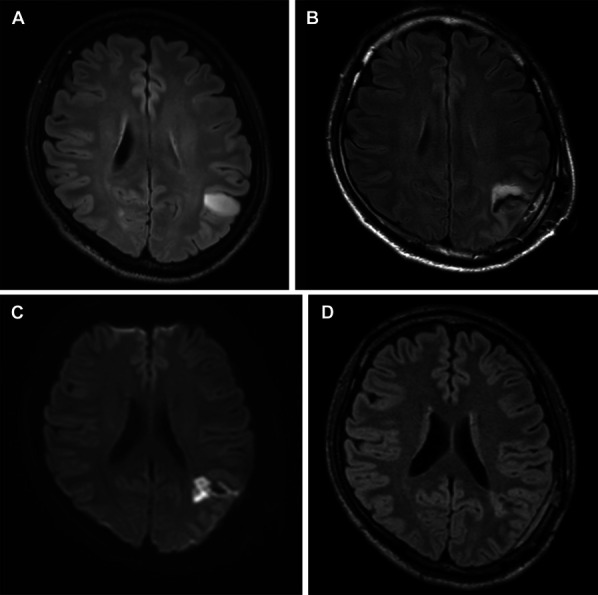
A, MRI of a 41-year-old asymptomatic man showing a left parietal brain tumor. B, Awake craniotomy to achieve gross total resection is performed (postoperative exudate fluid gives the appearance of high FLAIR signal but not residual tumor). Forty-eight hours after surgery, paraphasia appeared. C, DWI-MRI shows cerebral ischemia in the deep white matter; D, without a high-intensity area on FLAIR image corresponding to an ischemic lesion. FLAIR, fluid-attenuated inversion recovery.
